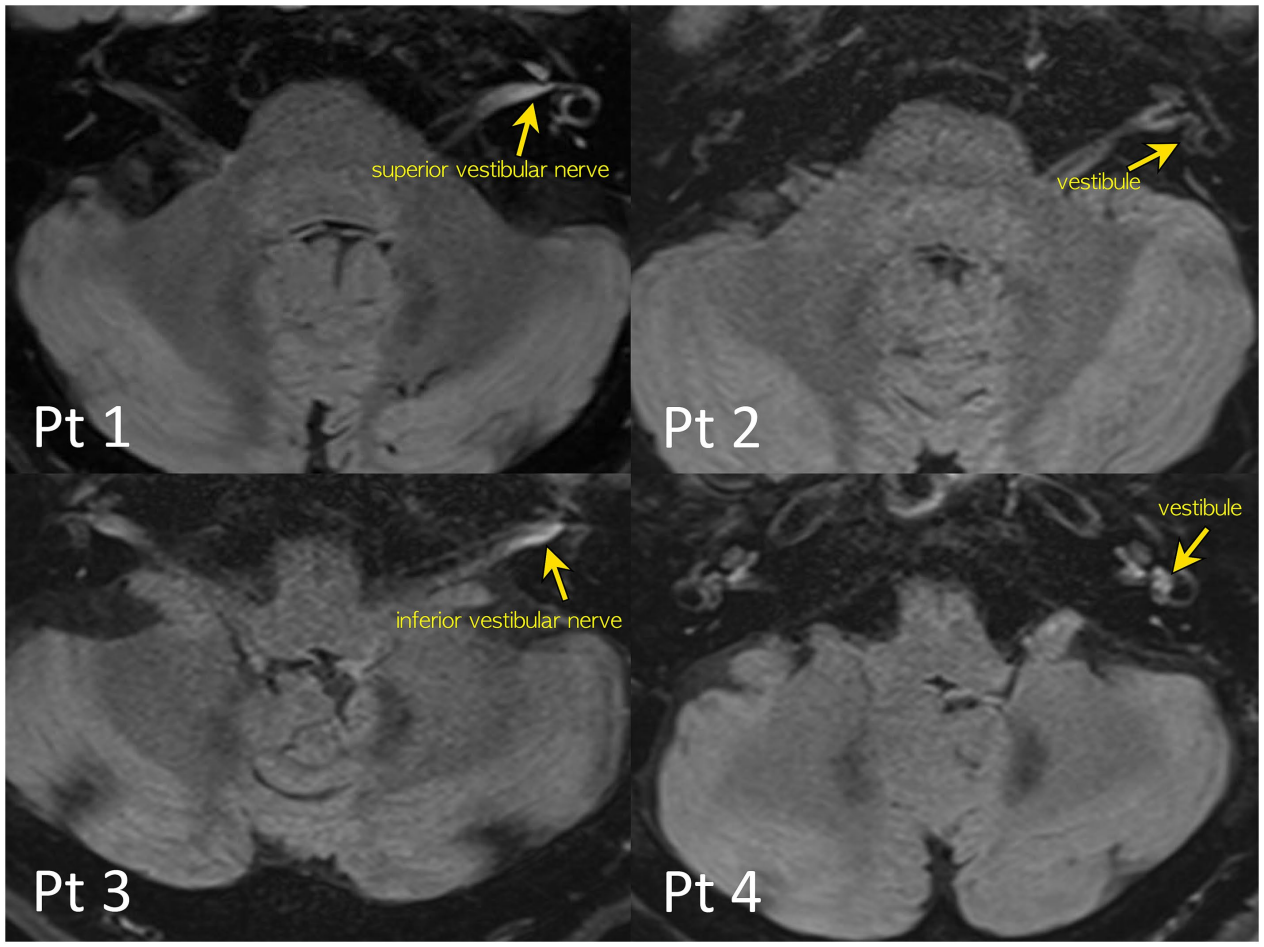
4-h delayed 3D-FLAIR images of the patients. Quantitative evaluation of a degree of the perilymphatic enhancement. The signal intensity ratios of the vestibular nerves and inner ear structure to that of the signal intensity of the medulla were calculated to avoid bias from patient-related artifacts.
